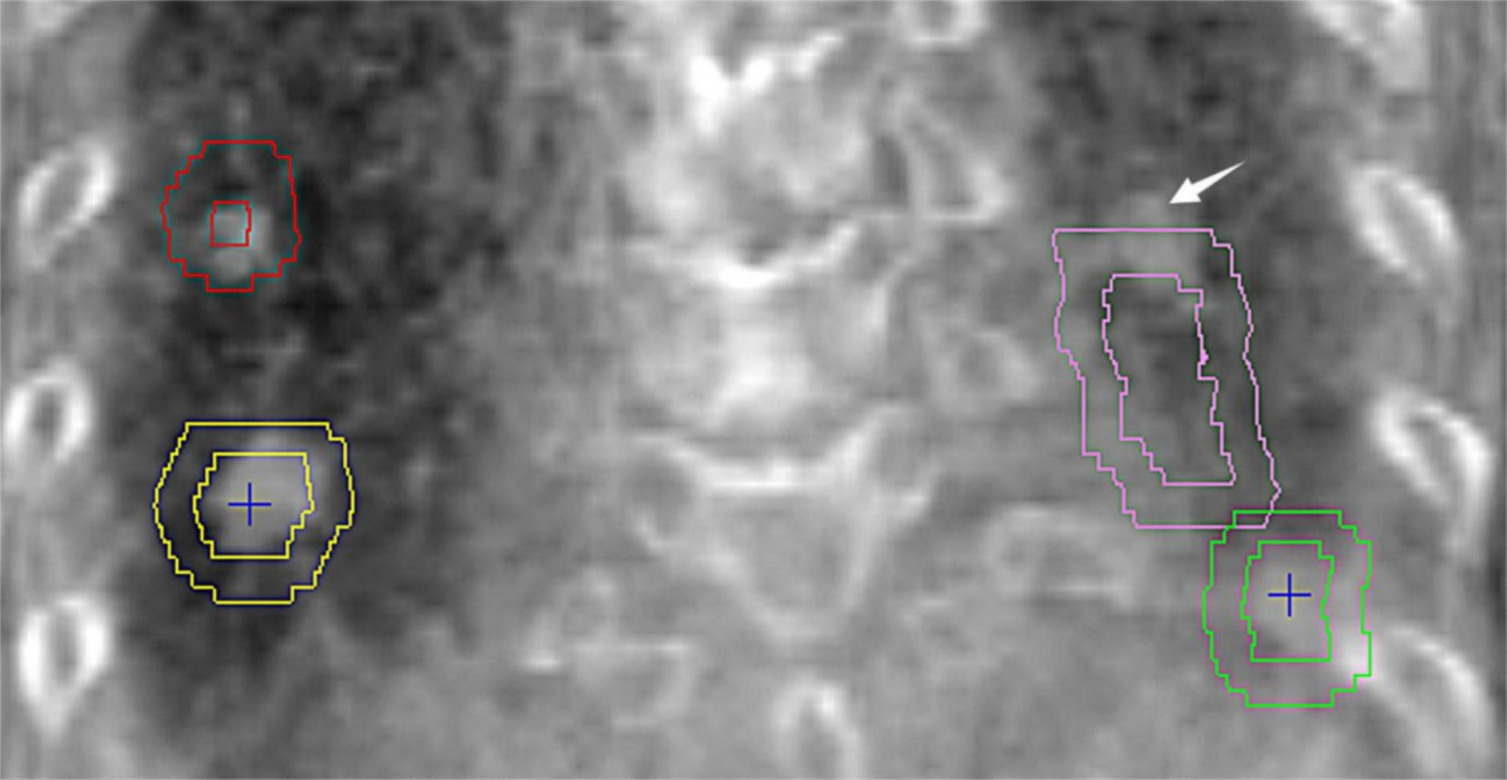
Example of a geometric miss in a patient with multiple tumors in the lower lobe. On the time-averaged CBCT image, 2 out of the 4 tumors reached the border of the PTV (red and yellow outer outlines) as contoured on the reference CT (AIP image), hindering the correction of tumor position, such that one of the tumors (arrow) exceeded the PTV
